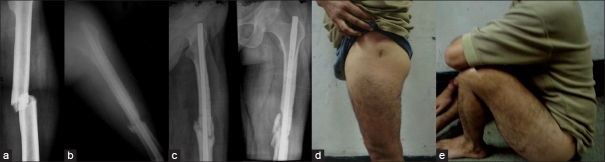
(a) Preoperative anteroposterior X-ray of thigh showing a transverse fracture soft femur. (b) Post-operative antero-posterior X-rays of thigh showing well aligned fracture with intramedullary expandable nail. (c) Anteroposterior and lateral view follow up X-rays showing fracture union. (d,e) clinical result at final follow up showing good functional outcome
